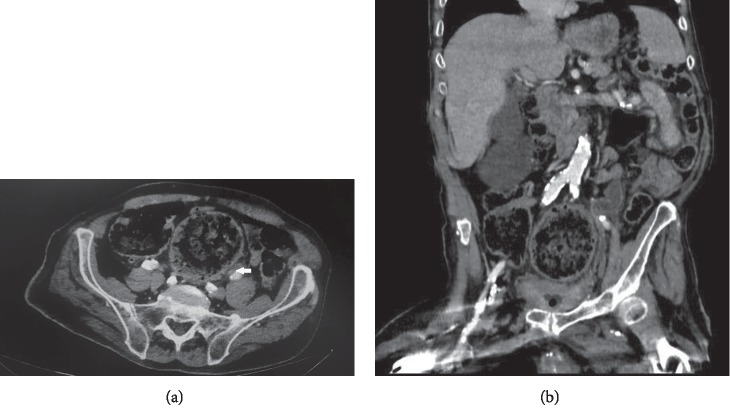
Computerized tomographic (CT) scan of the abdomen and pelvis ((a): axial view, (b): coronal view). Extrinsic compression of the left midureter (arrow) between an atherosclerotic external iliac artery and a giant fecaloma.
